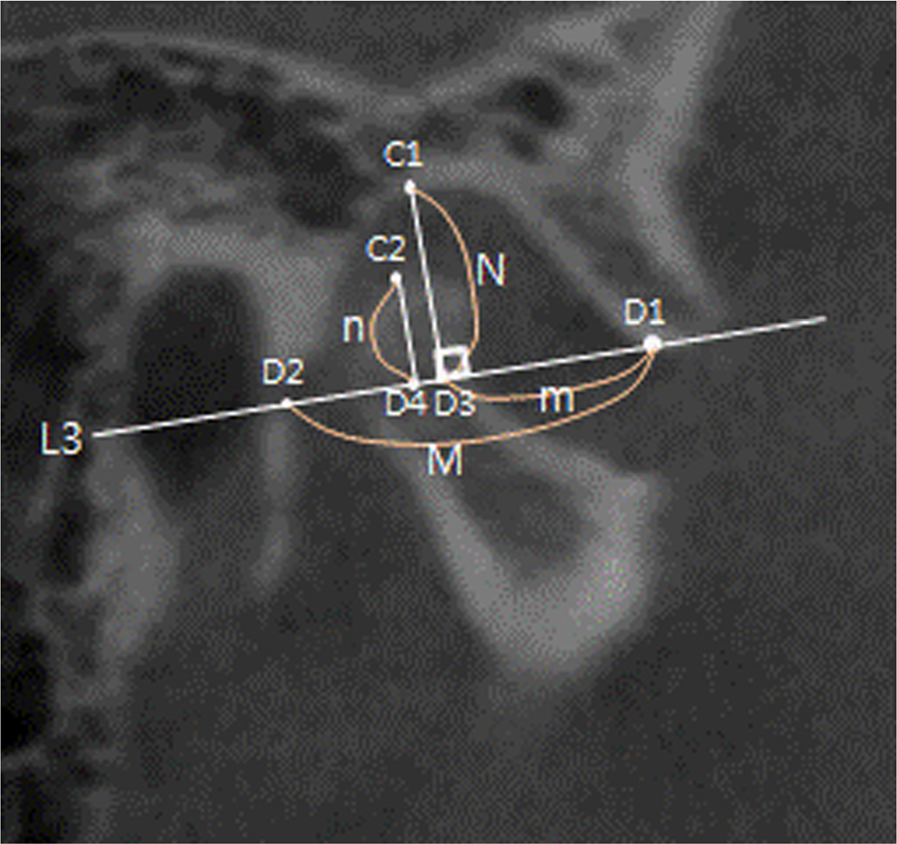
Reference points and measurement of sagittal view in CBCT. C1 highest point of glenoid fossa, C2 highest point of condylar head, D1 lowest point of articular eminence, D2 lowest point of temporal squamotympanic fissure, L3 line passing D1 and D2, D3 point meeting the perpendicular line from C1 on L3, D4 point meeting the perpendicular line from C2 to L3, *M* distance between D1 and D2, *m* distance between D1 and D3, *N* distance between C1 and D3, *n* distance between C2 and D4, S–N *n*/*N* (assessed by ratio of vertical location of mandibular condyle at glenoid fossa), S–M, *m*/*M* (assessed by ratio of horizontal location of mandibular condyle at glenoid fossa)
